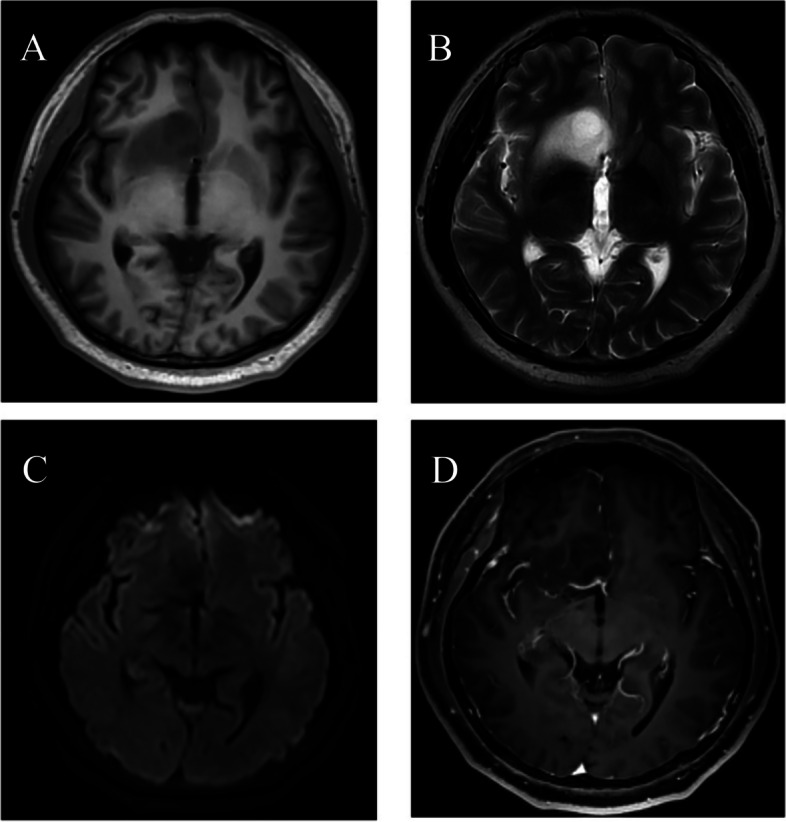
33-year-old male patient with type A anterior perforated substance (APS) glioma. The lesion involves the right posterior part of straight gyrus, the anterior and inferior part of basal ganglia, and the medial of insula. It is low signal on T1-weighted image (T1WI) (A), high signal on T2-weighted image (T2WI) (B), low signal on diffusion weighted image (DWI) (C), and no enhancement (D)
